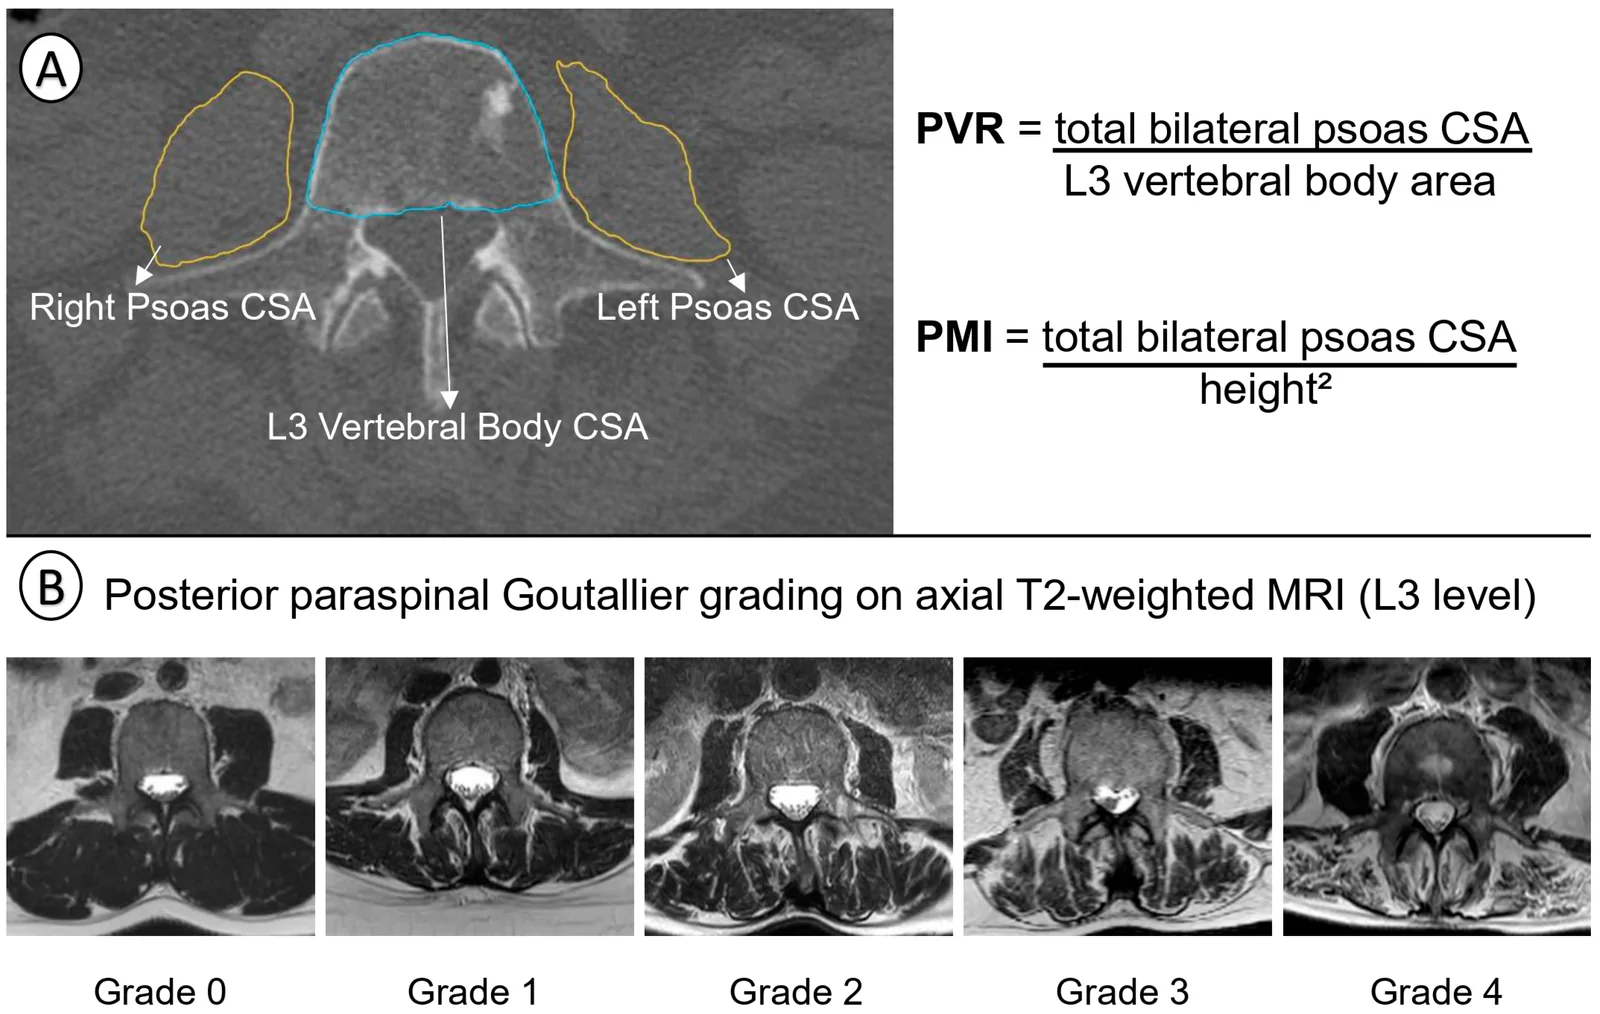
Imaging assessment of posterior paraspinal fatty infiltration and the exploratory combined axial muscle phenotype. (A) Example of L3 CT-based psoas assessment showing bilateral psoas cross-sectional area and L3 vertebral body cross-sectional area measurements, used to calculate the psoas-to-vertebral-body ratio and psoas muscle index. (B) Representative axial T2-weighted L3 MRI examples of posterior paraspinal Goutallier grades 0–4 in the multifidus/erector spinae compartment. For primary analyses, patient-level maximum posterior paraspinal Goutallier grade was dichotomized as low-grade fatty infiltration, grades 0–2, versus high-grade fatty infiltration, grades 3–4. CSA, cross-sectional area; MRI, magnetic resonance imaging; PVR, psoas-to-vertebral-body ratio; PMI, psoas muscle index.
